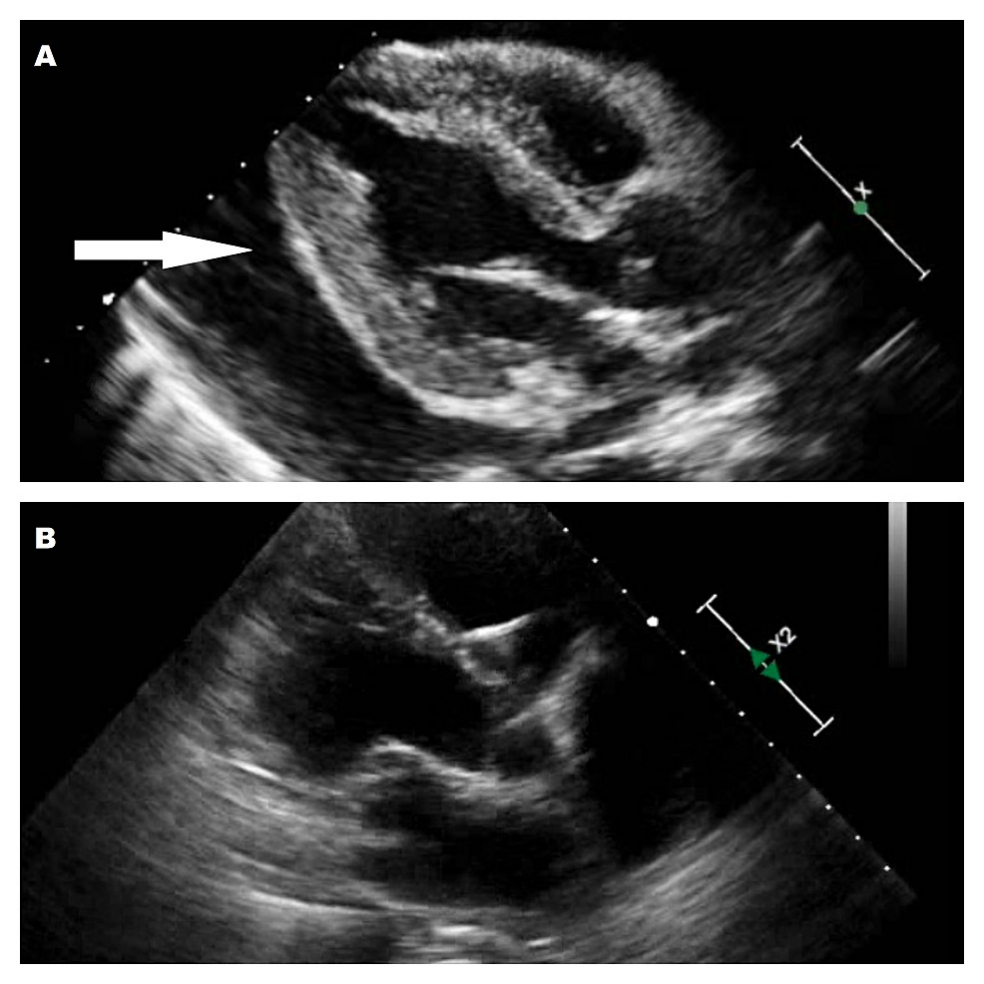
(A) Initial echocardiogram showing large pericardial effusion (white arrow) and (B) Post pericardiocentesis echocardiogram showing resolution of Pericardial effusion
